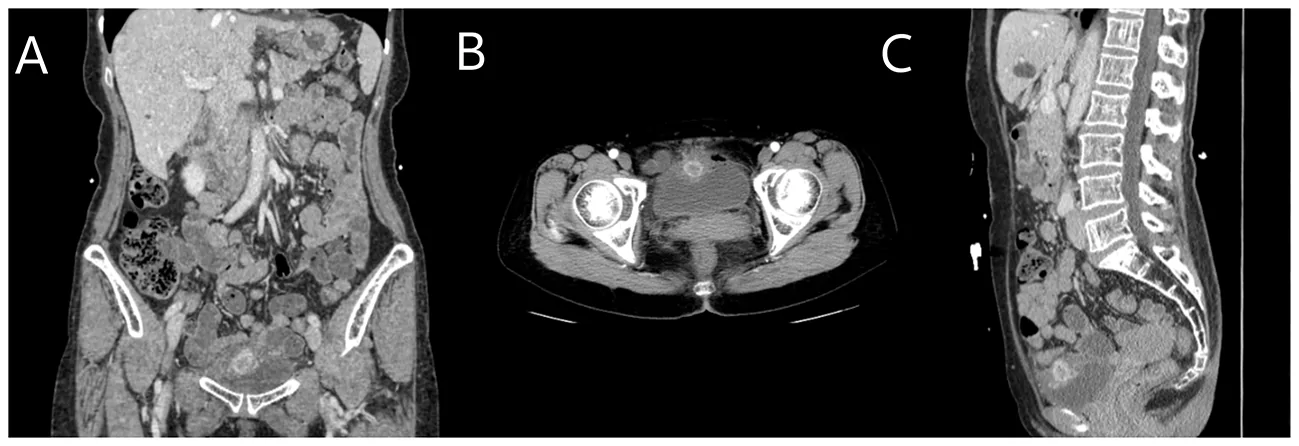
Preoperative contrast-enhanced CT of urachal inflammatory myofibroblastic tumor. (A) Coronal view; (B) Axial view; (C) Sagittal view. A well-defined soft-tissue mass was located in the midline of the urachal region.
